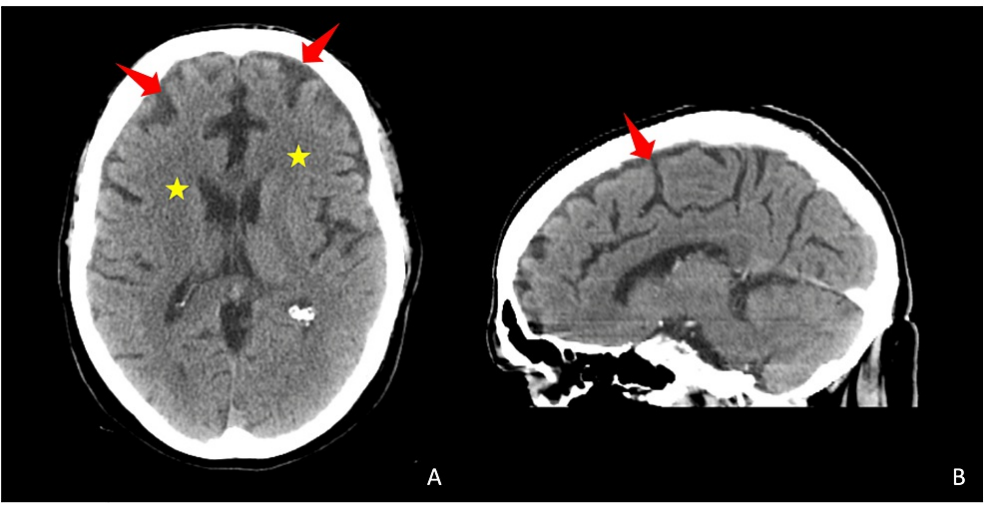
Transverse (A) and sagittal (B) slices obtained from computed tomography (CT) of the head with intravenous contrast. Global brain atrophy (arrow) and chronic ischemic changes (star) are notable in this scan. There is no evidence of an acute intracranial process.
